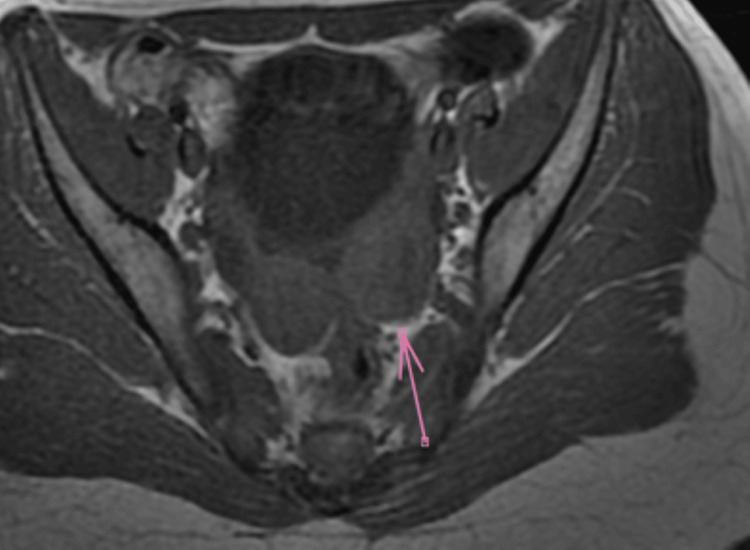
Axial pelvic MRI in T1-weighted sequence showing the presence of a mass classified as O-RADS 4 (arrow), highly suspicious for malignancy. O-RADS: Ovarian-Adnexal Reporting and Data System.
